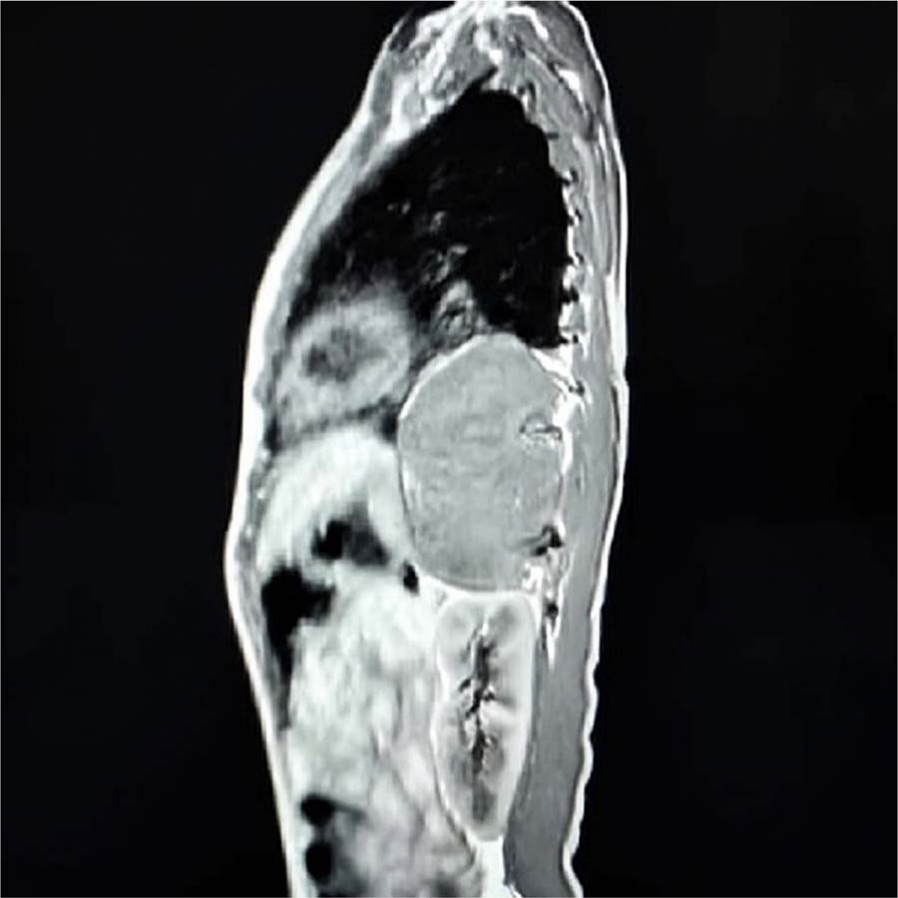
Sagittal contrast-enhanced CT scan showing a well-defined, homogeneous, soft-tissue mass (11 × 7 × 5 cm) in the left posterior mediastinum, abutting the descending aorta and thoracic vertebrae.
